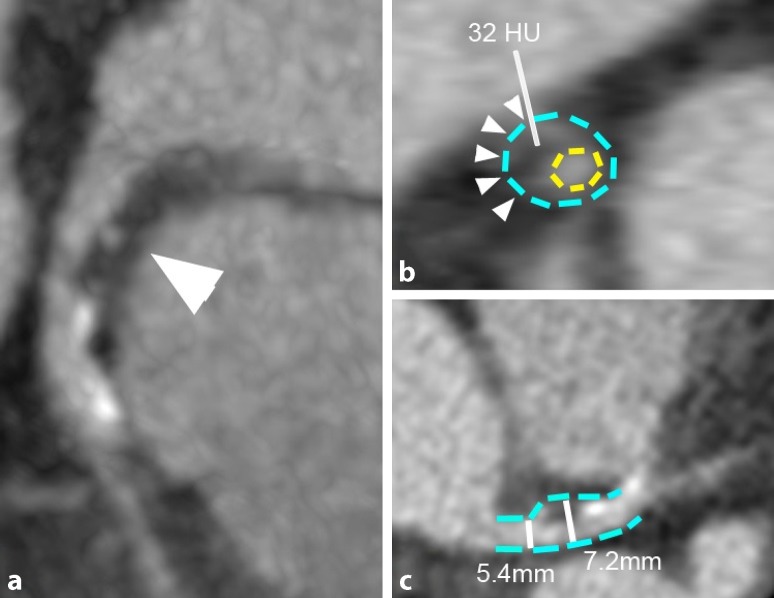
A 61-year-old patient with a history of smoking and diabetes. CTA revealed four high-risk plaque features in the distal left main/proximal left anterior descending artery (a *arrowhead*): low-attenuation plaque (b *annotation*) with napkin-ring sign (b *arrowheads*) and positive remodelling (b, c *dashed line*) and spotty calcification (a, c). The patient suffered from non-ST elevation myocardial infarction 2 months after CT and died of heart failure 3 years later
